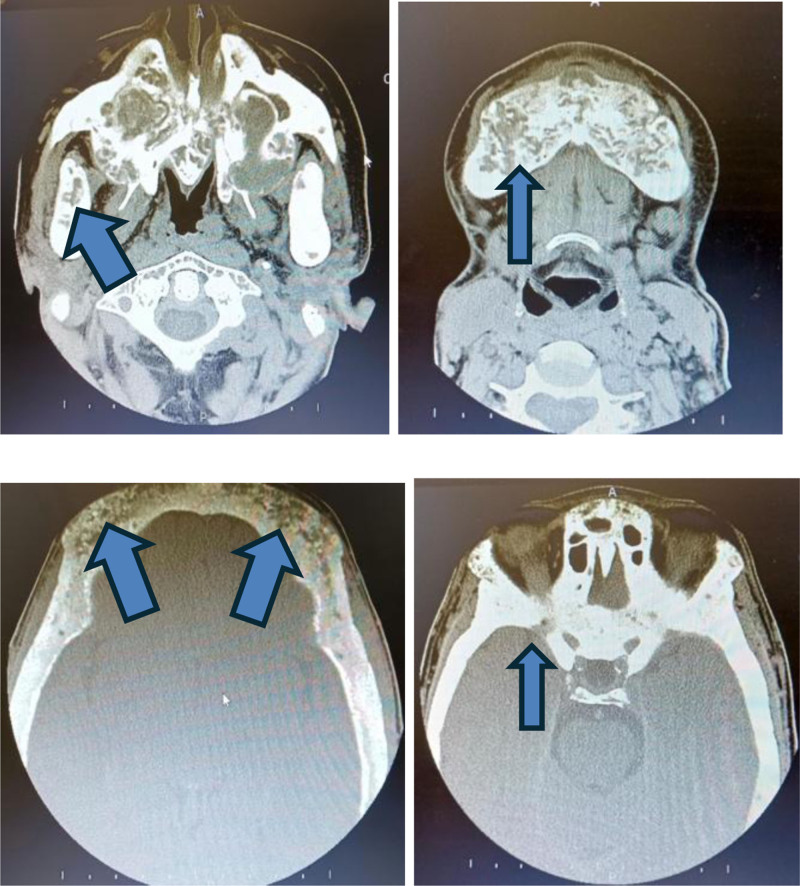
CT paranasal sinuses – FESS protocol showing lytic lesions of skull and facial Bones pointed by blue arrows. CT = computed tomography, FESS = functional endoscopic sinus surgery.
